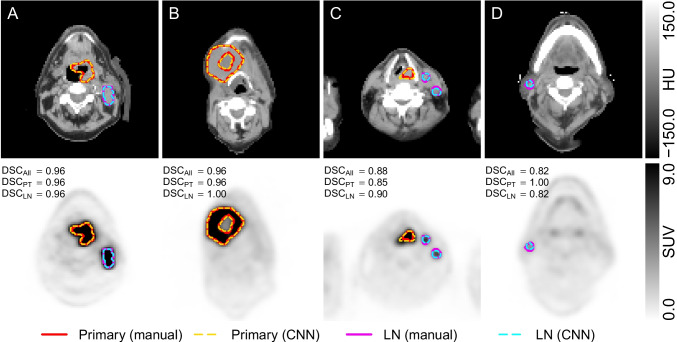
Manual and CNN-based delineations of primary tumor and lymph node metastases in 4 selected patients. Relevant transaxial PET/CT slices are shown (top: CT, bottom: PET). The dice coefficients (in the presented plane) for primary tumor ($$\text {DSC}_{\text {PT}}$$), LN metastases ($$\text {DSC}_{\text {LN}}$$), and their union ($$\text {DSC}_{\text {All}}$$) are indicated. Patient A: oropharyngeal cancer with LN metastasis; patient B: oropharyngeal cancer with necrotic core; patient C: hypopharyngeal cancer with 2 LN metastases; patient D: cancer of oral cavity (not visible in this slice) exhibiting a low uptake LN metastasis
